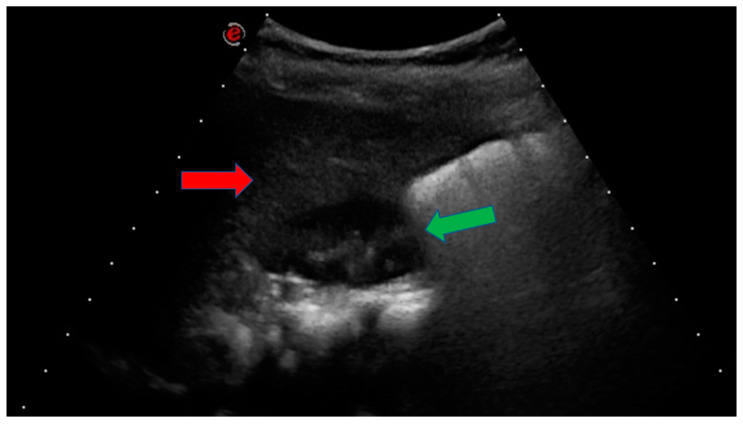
The proband’s abdominal ultrasonography: the liver (red arrow) appears hyperechogenic compared to the kidney (green arrow).
